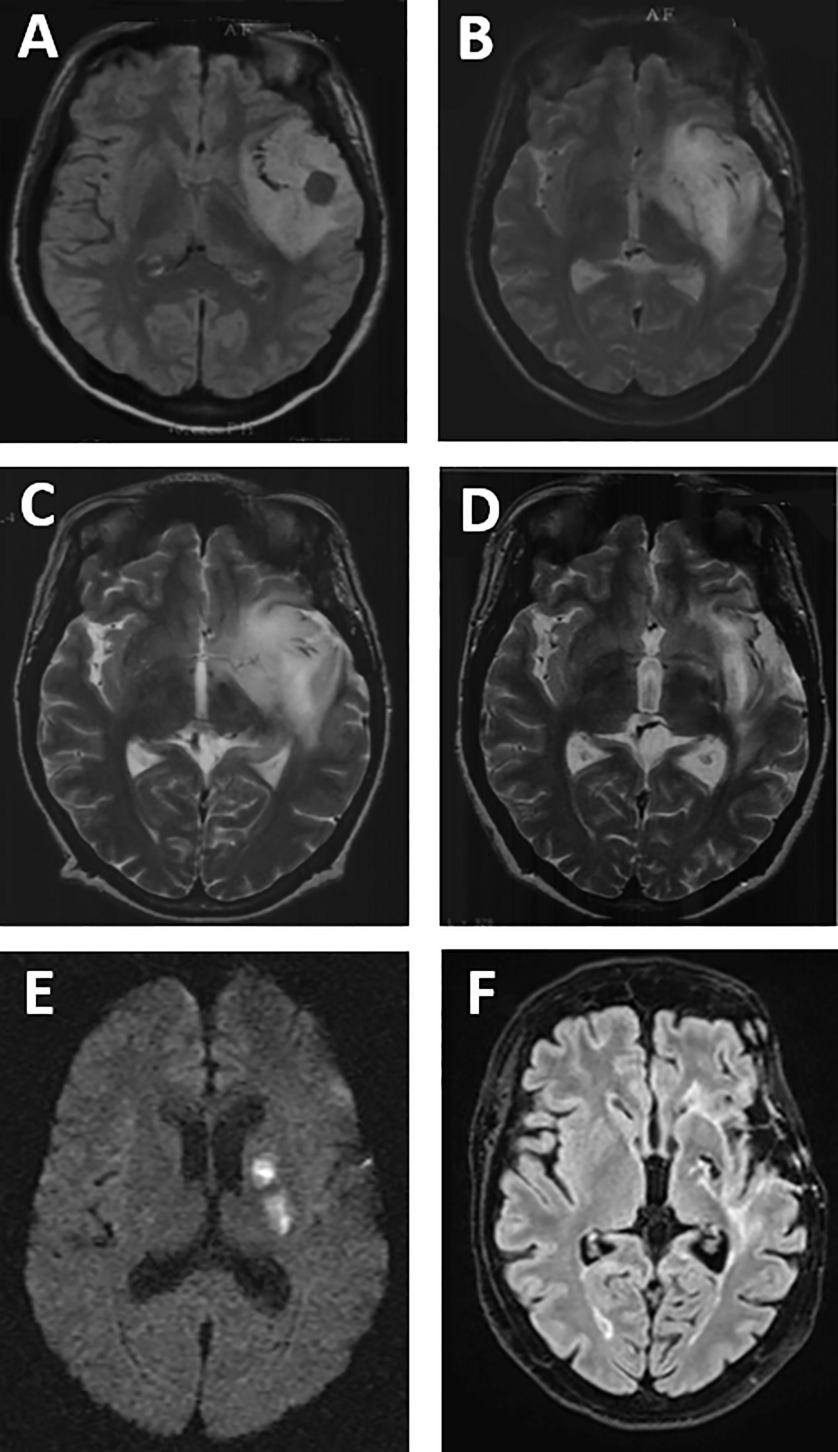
Example of MRI response and complication, in left temporal IDH/noncodeleted DLGG, in FLAIR (A, F), T2-weighted (B–D), and diffusion-weighted imaging (E). Precise (A) at diagnosis and after biopsy. (B) MRI before PCV and 3 months after partial resection. (C) MRI after PCV (before RT). (D) MRI after RT (after PCV + RT). (E) MRI 6 years after the end of PCV + RT (stroke lesions). (F) MRI 19 years after the end of PCV + RT and 25 years after diagnosis.
